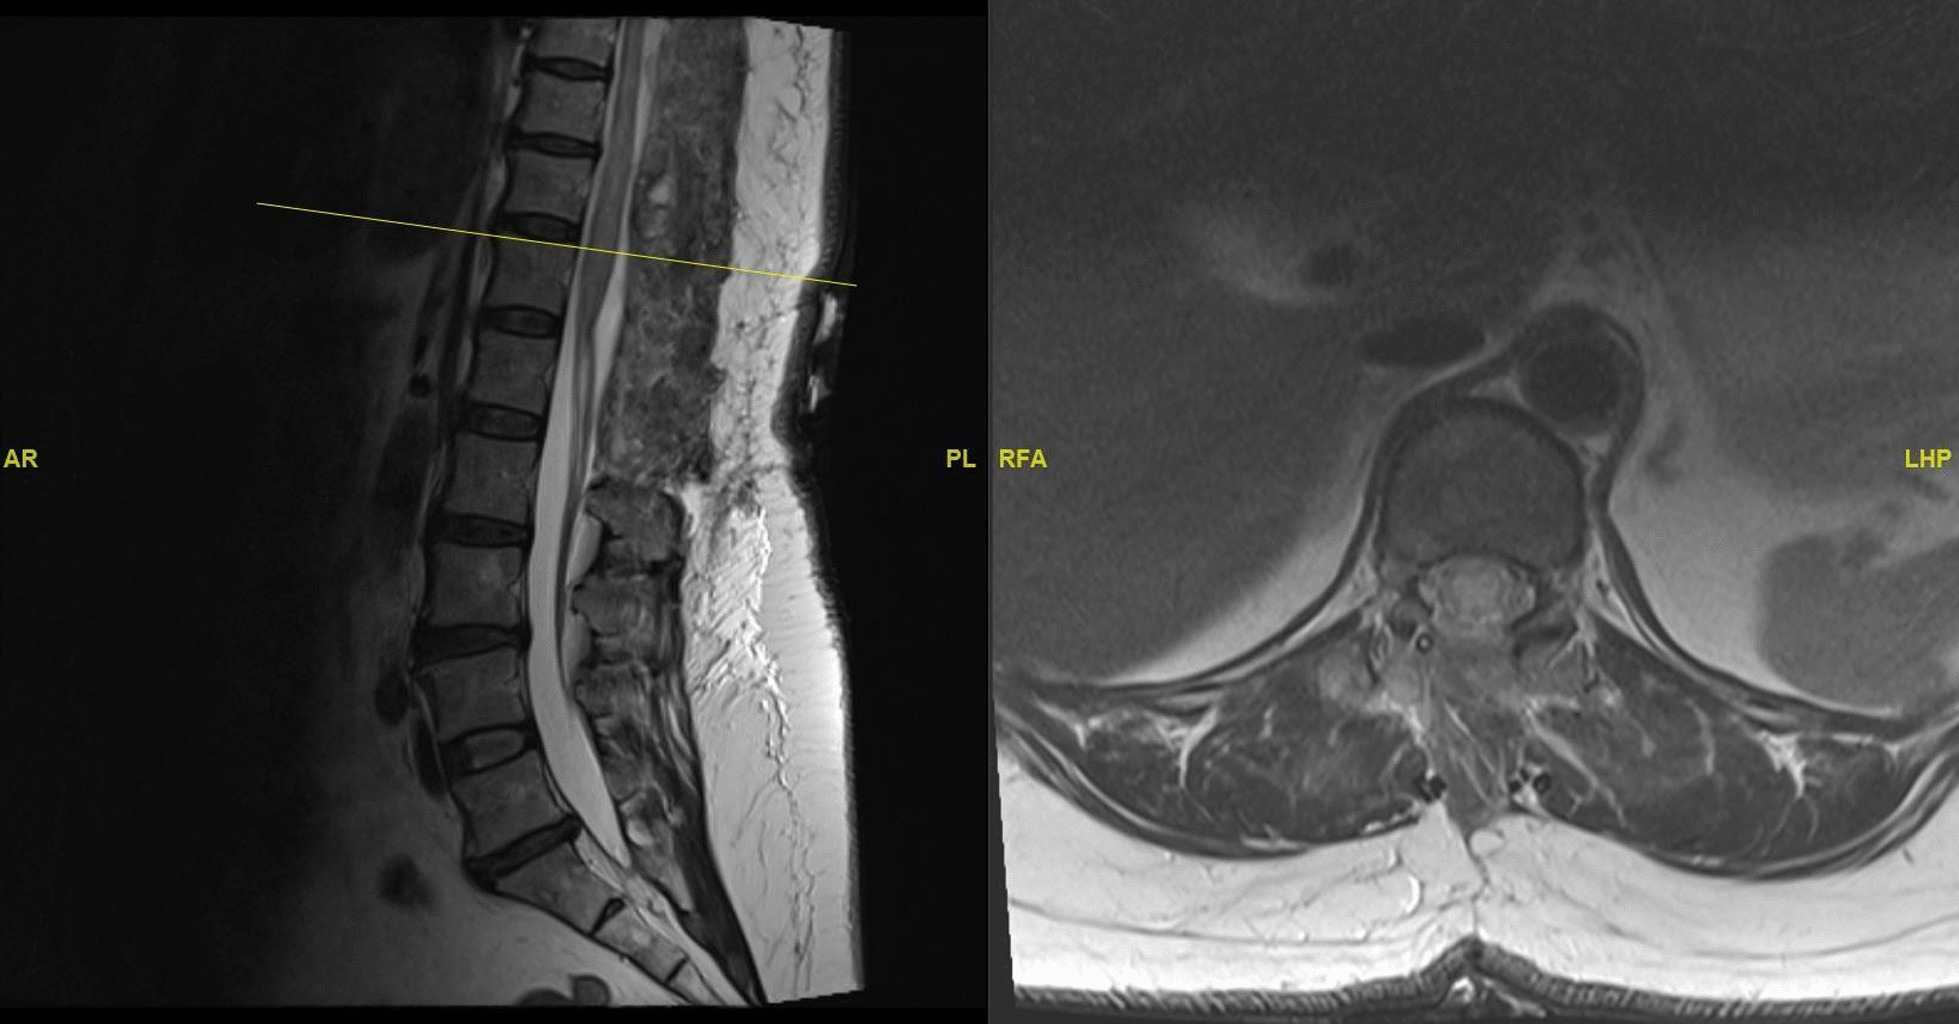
Post-operative MRI whole spine
